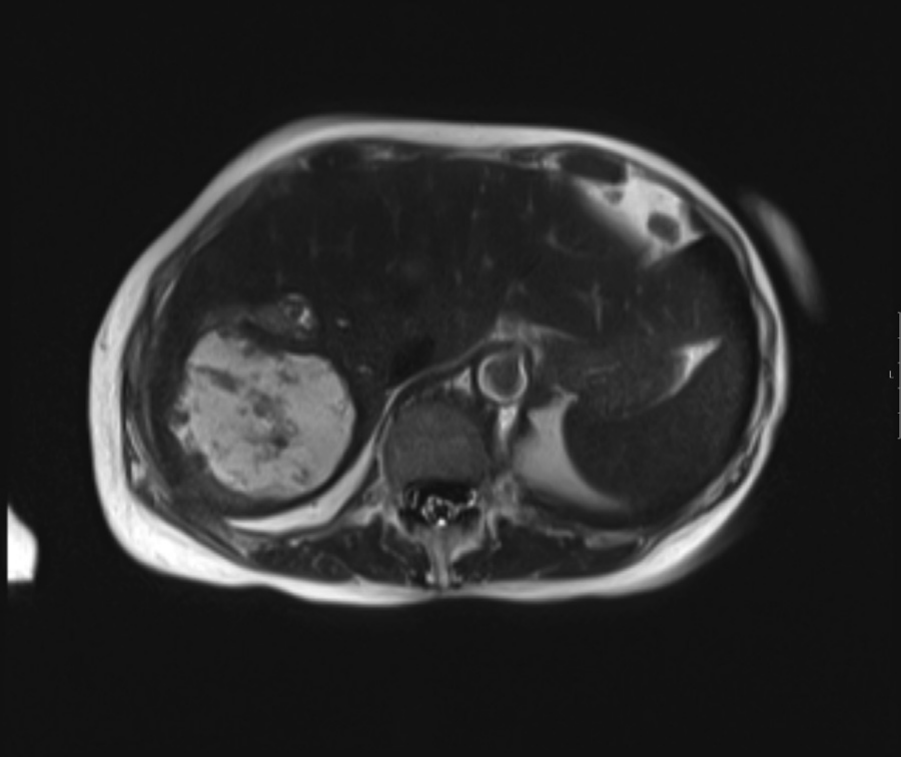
T2W magnetic resonance imaging of the liver demonstrating high signal fluid collection within the treated area of the liver.
